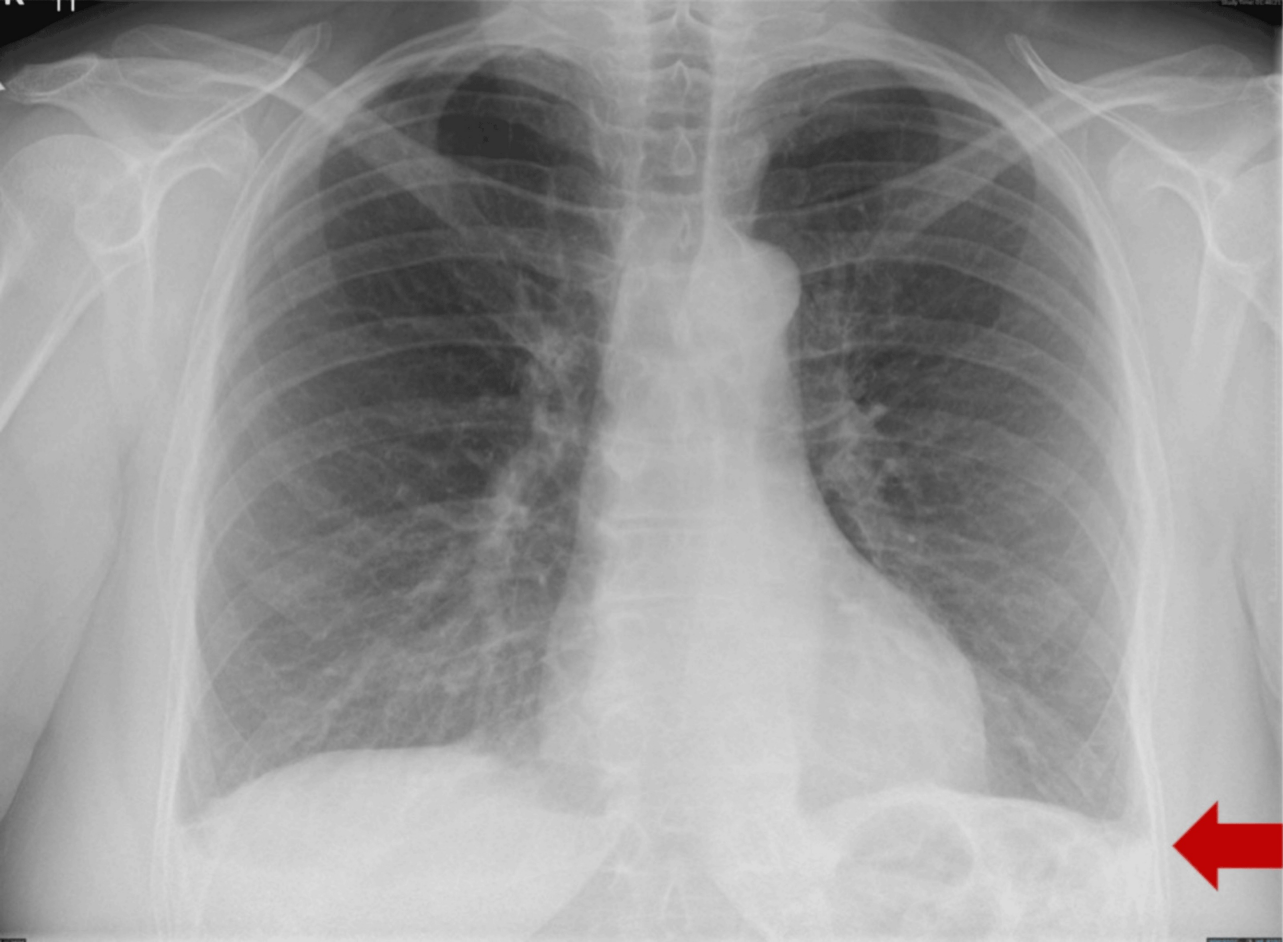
Chest X-ray posteroanterior view showing a small left-sided pleural effusion (red arrow).
